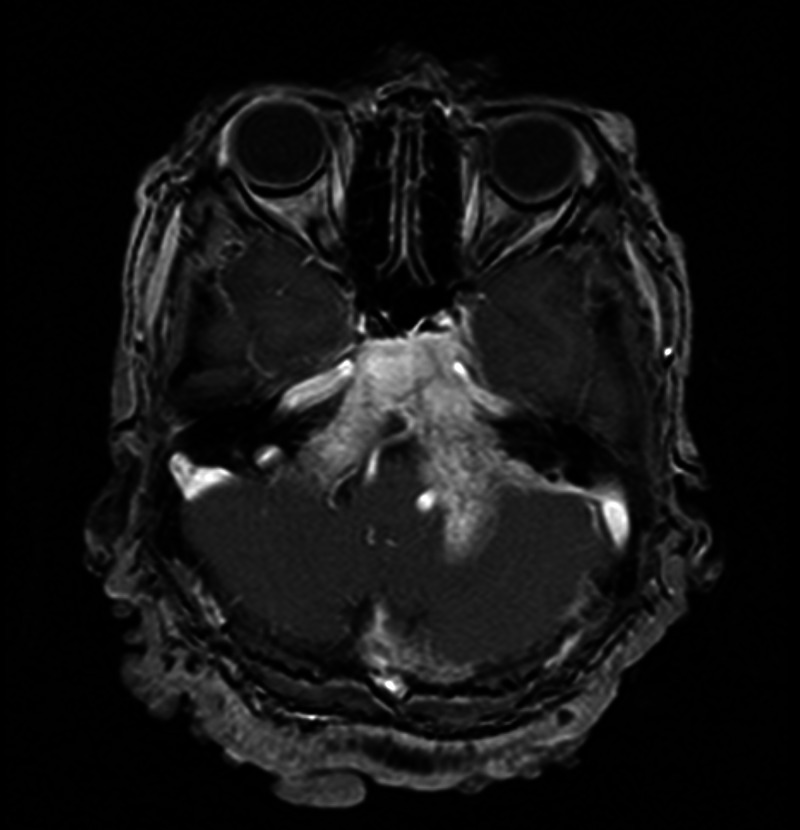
Magnetic resonance imaging scan of the brain showing destructive skull bone lesions along with compressing effect on the pons and the medulla oblongata.
